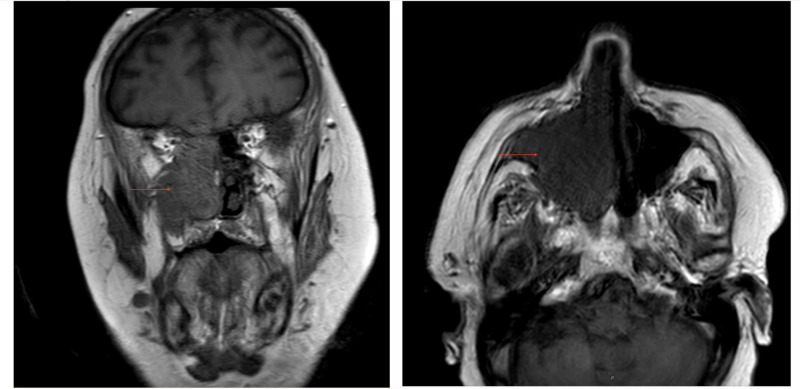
Case one magnetic resonance imaging face with and without contrast Left: coronal plane; red arrow points to mass; Right: axial plane; red arrow points to mass
